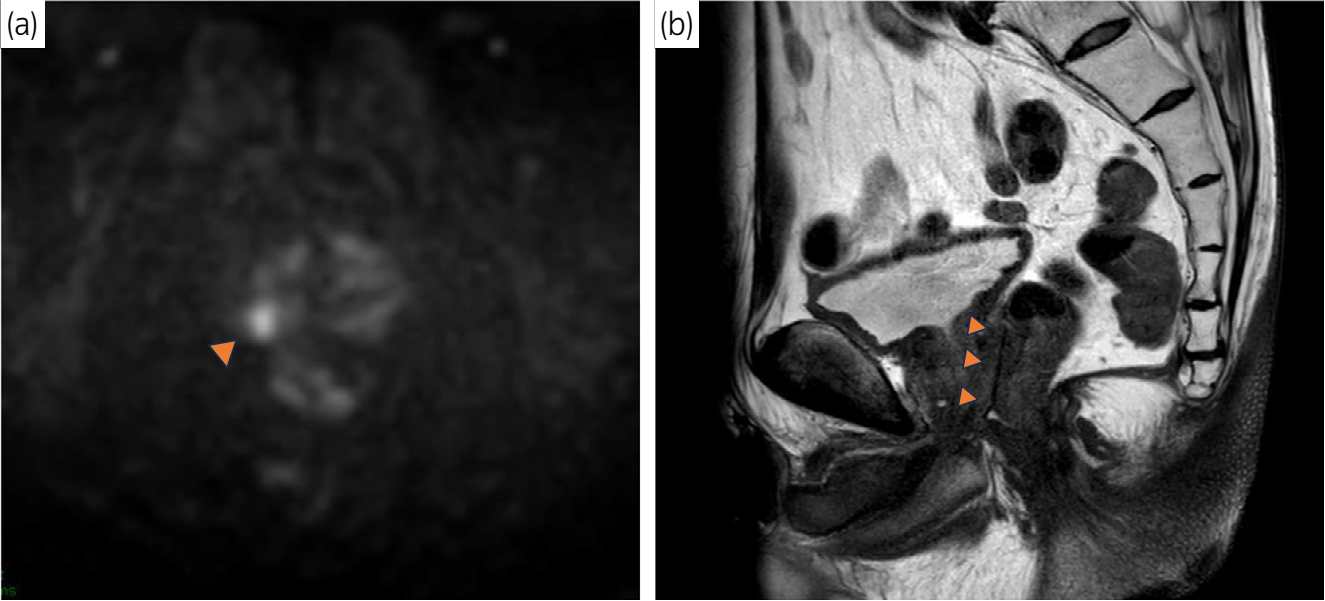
(a) Axial diffusion‐weighted MRI revealed prostate cancer lesion with a PI‐RADS category 4 in the right peripheral zone of the prostate. (b) Sagittal T2‐weighted MRI showed the prostate and its dorsal bowel were in contact, with no fat in between.
